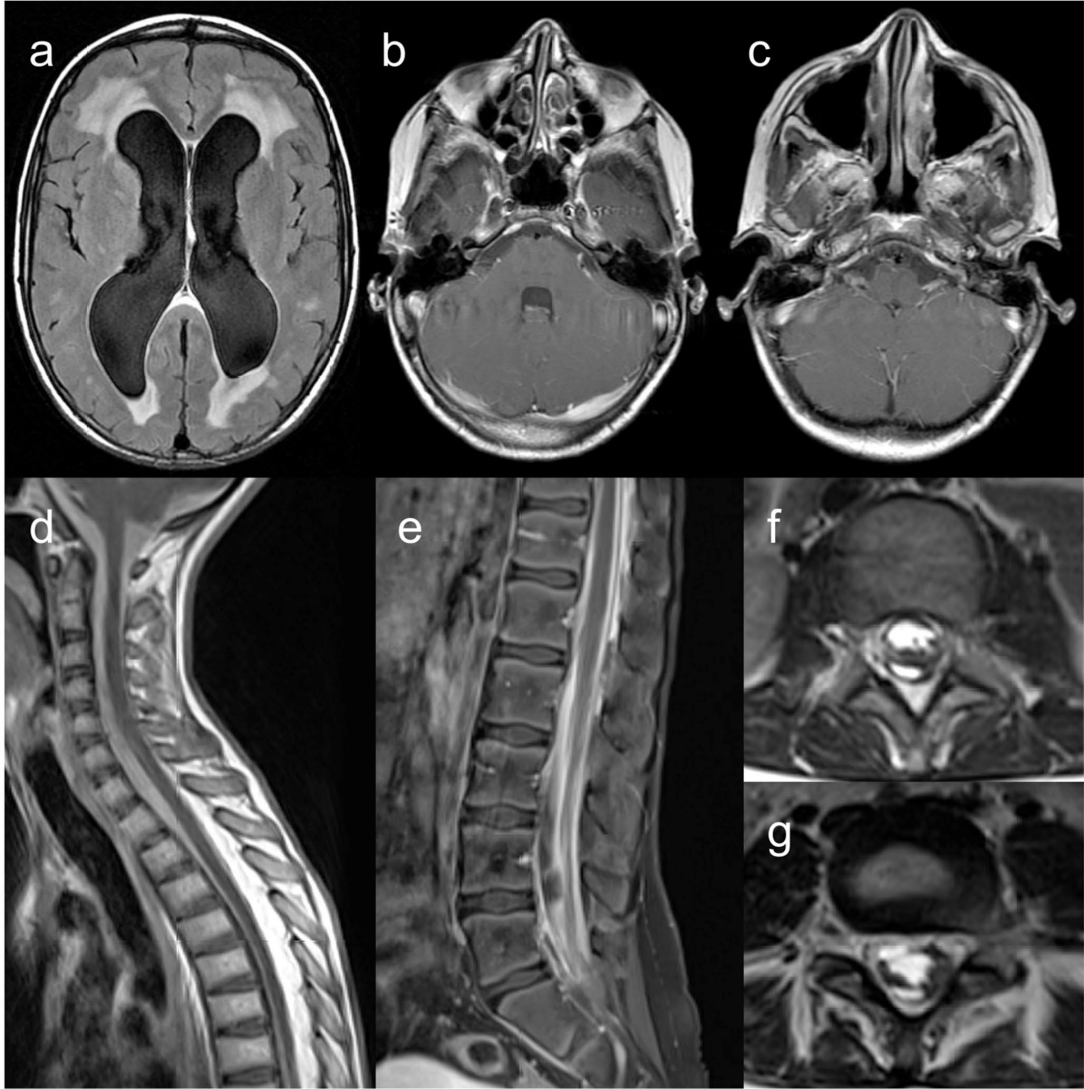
X-linked Agammaglobulinemia. A 16-year-old female patient with chronic enteroviral meningitis associated with congenital agammaglobulinemia. Brain MRI (a – axial FLAIR, and b and c – gadolinium-enhanced axial T1) shows chronic supratentorial hydrocephalus, which is slightly more pronounced compared to previous scans (not shown). There is concomitant transependymal edema, partially confluent with multifocal T2-hyperintense white matter lesions. Leptomeningeal enhancement is observed surrounding the brainstem, involving some cranial nerves, and extending into the internal auditory canals. Spine MRI (d and e – gadolinium-enhanced sagittal T1, and f and g – gadolinium-enhanced axial T1 at two lumbar levels) reveals features suggestive of extensive spinal pachymeningitis and arachnoiditis
